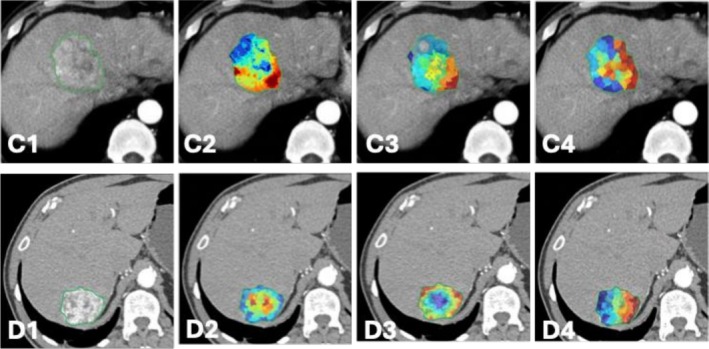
(C) A 58‐year‐old male patient with moderately differentiated HCC. The patient received transarterial chemoembolization and developed recurrence 21 months later. (C1) Arterial‐phase CT with lesion delineation. (C2) Nested habitat analysis results. (C3) Intensity‐based k‐means clustering results. (C4) Super pixel clustering results. (D) A 71‐year‐old male patient with moderately differentiated HCC. The patient received IMRT at a total dose of 54 Gy. (D1) Arterial‐phase CT with lesion delineation. (D2) Nested habitat analysis results. (D3) Intensity‐based k‐means clustering results. (D4) Super pixel clustering results.
